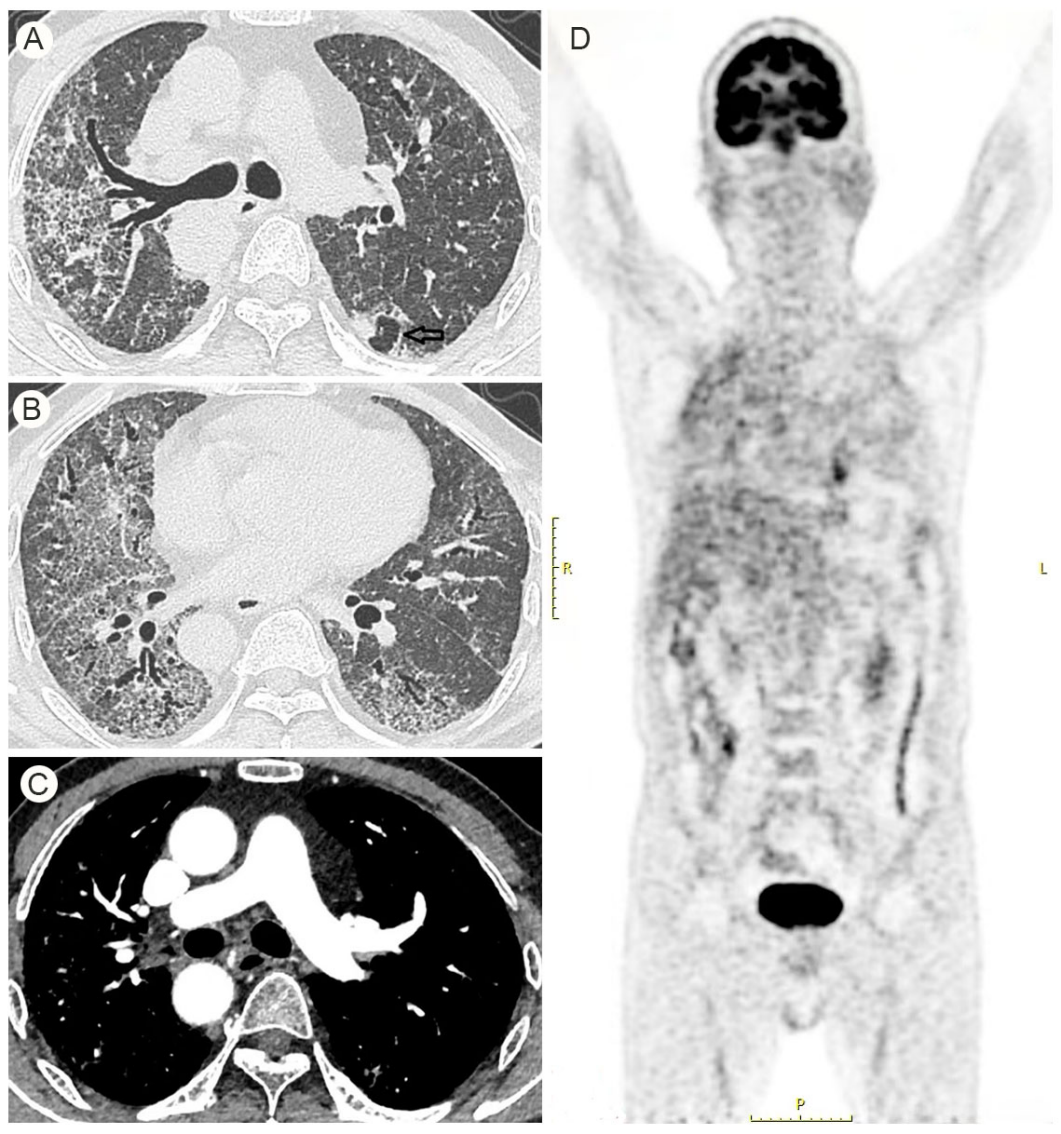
Preoperative HRCT signs: (A, B) diffuse ground-glass shadows in both lungs, dilated fine bronchioles, reticular shadows, striated lines and stretched bronchioles were seen in the proximal pleura, volume reduction in the lower lungs bilaterally. (C) Right aortic arch. (D) Foci of fibrosis in both lungs, foci of calcification in both lungs, localised thickening of the pleura in both lungs, inflammatory enlarged lymph nodes in the mediastinum.
